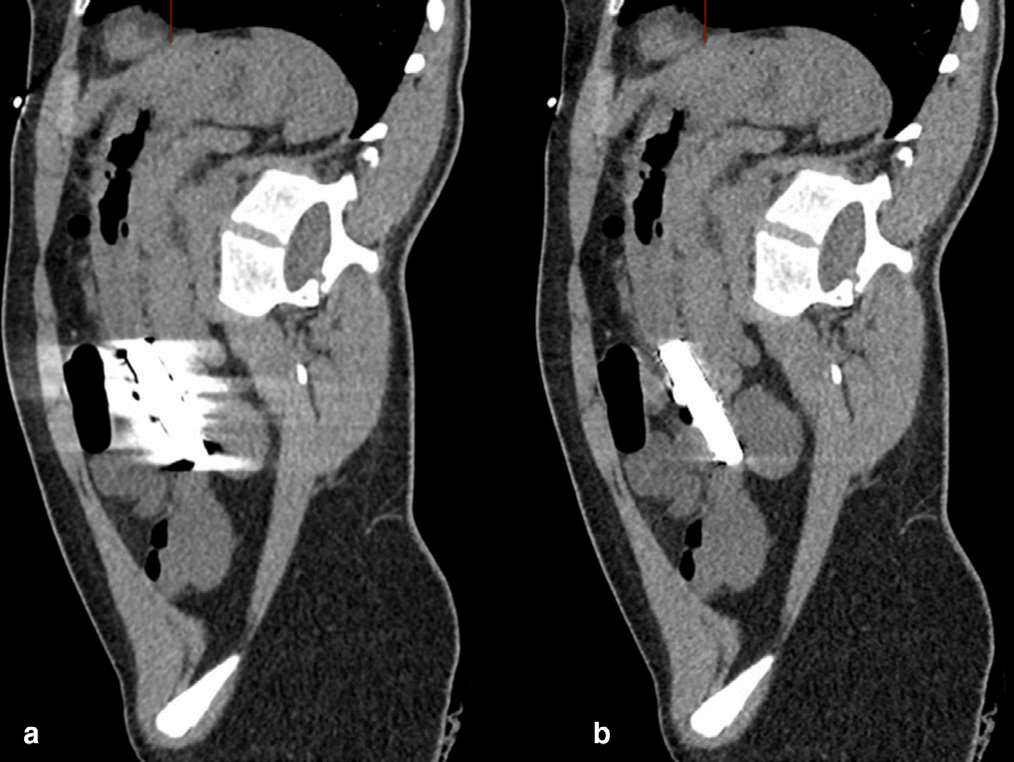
a,b: Sagittal oblique MPR CT image in the plane of the magnets, demonstrating the aggregation of button magnets. (a) No metal reduction artefact applied. (b) Metal reduction artefact applied.
